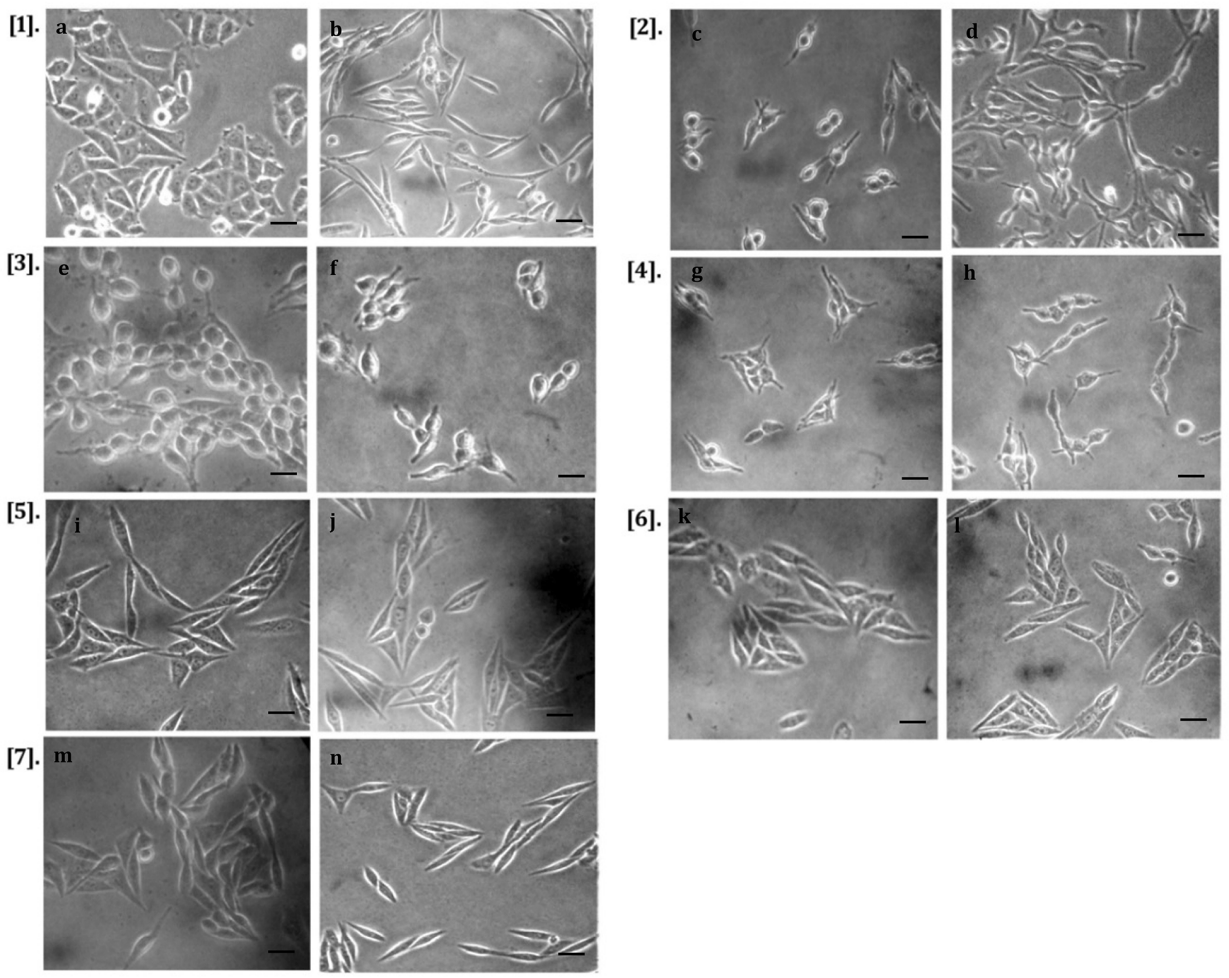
Protective activity against CT-induced cell elongation in CHO cell line. [1] (A) CHO cell control, (B) CFCF control (CT=100ng/mL); [2] (C) RTN (NC1)+CFCF, (D) RTN (NC2)+CFCF; [3] (E) PHD (NC1)+CFCF, (F) PHD (NC2)+CFCF; [4] (G) QRTN (NC1)+CFCF, (H) QRTN (NC2)+CFCF; [5] (I) EA (NC1)+CFCF, (J) EA (NC2)+CFCF; [6] (K) CHL (NC1)+CFCF, (L) CHL (NC2)+CFCF; [7] (M) GA (NC1)+CFCF, (N) GA (NC2)+CFCF at 40X magnification (scale bar 5µm).
